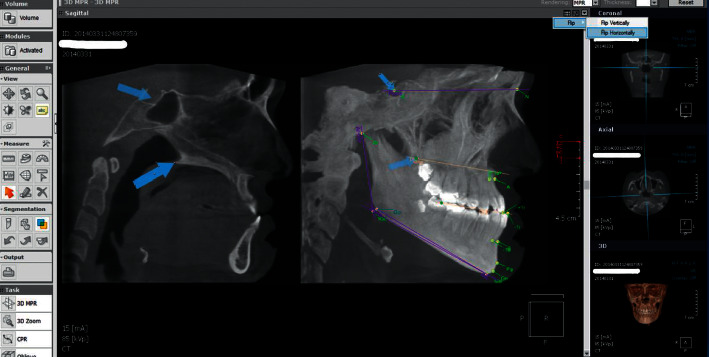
Left image: Two arrows were used to point out the exact places of points S (Sella) and PNS (Posterior Nasal Spine) and these arrows were preserved during slice manipulation. Right image: The thickness of the sagittal view was increased to 100 mm to get a CBCT-derived cephalometric image while keeping the arrows stable in their places in order to help in identifying points S and PNS accurately.
